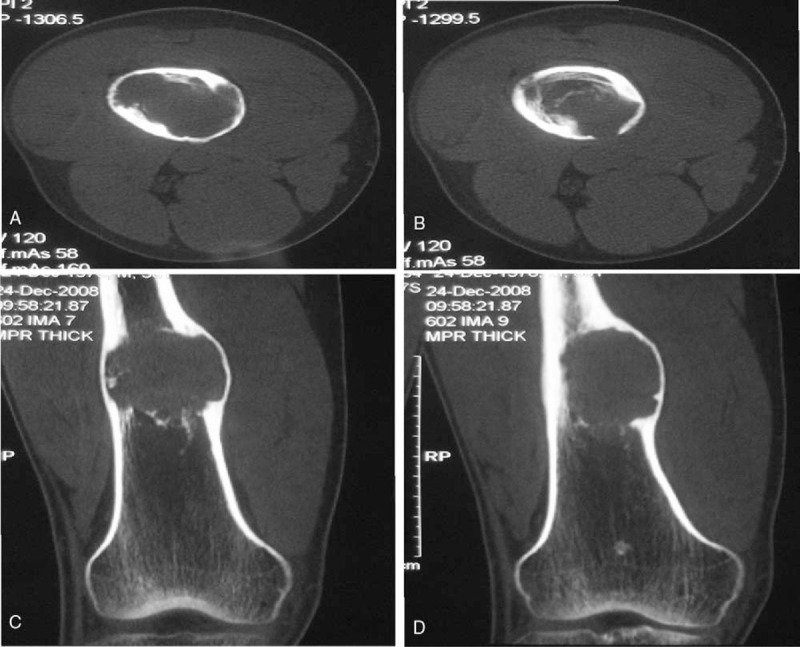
Computed tomography (CT) findings. Transaxial CT (A&B) and multiple plane reconstruction (C&D) demonstrated an expansive bone destruction region in the femur, with endosteal erosion, inner bone trabeculation, periosteal reaction, and posterial cortical disruption. Spotted calcification and “ground-glass-like” density are shown within the lesion. Average CT values in the lesion were 67-70Hu.
